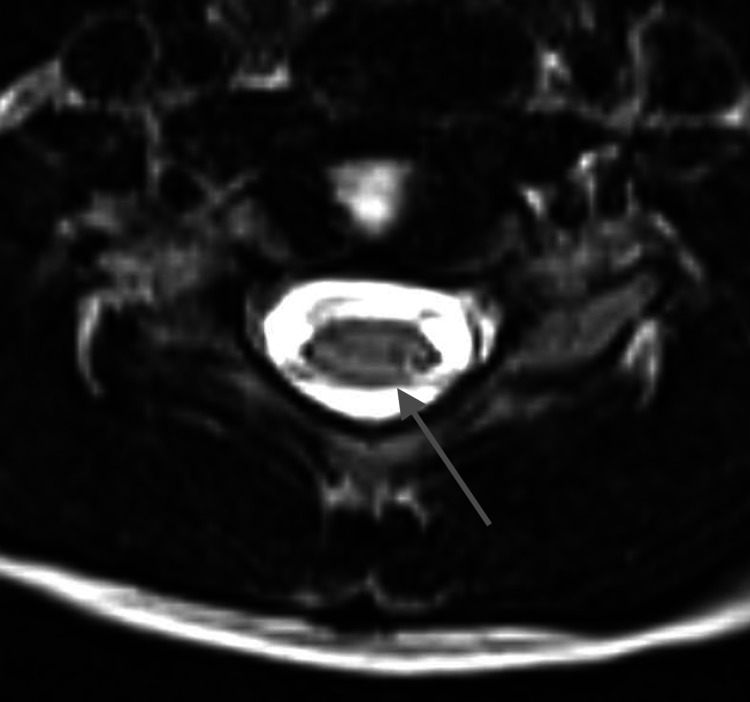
Axial T2-weighted image of the spine at the level of C6-7 at eight years of age shows resolution of the slit-like syrinx (gray arrow).
